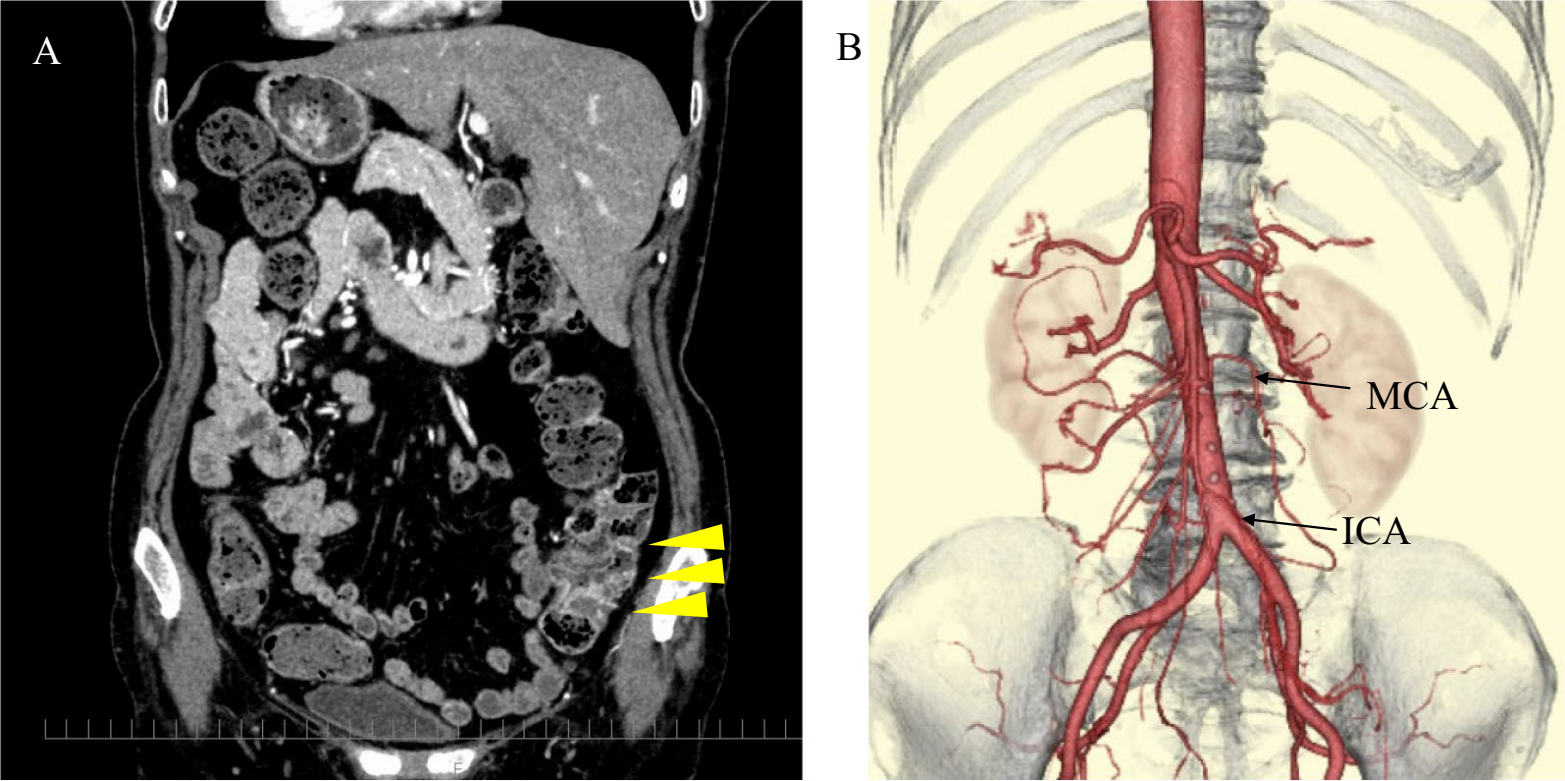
Abdominal CT and 3D-CT angiography. A CT revealed SIT and ascending colon cancer (yellow triangle) but no lymph node metastasis or distant metastasis. B CT angiography showed the ileocecal and middle colic artery. No anomalies were noted
